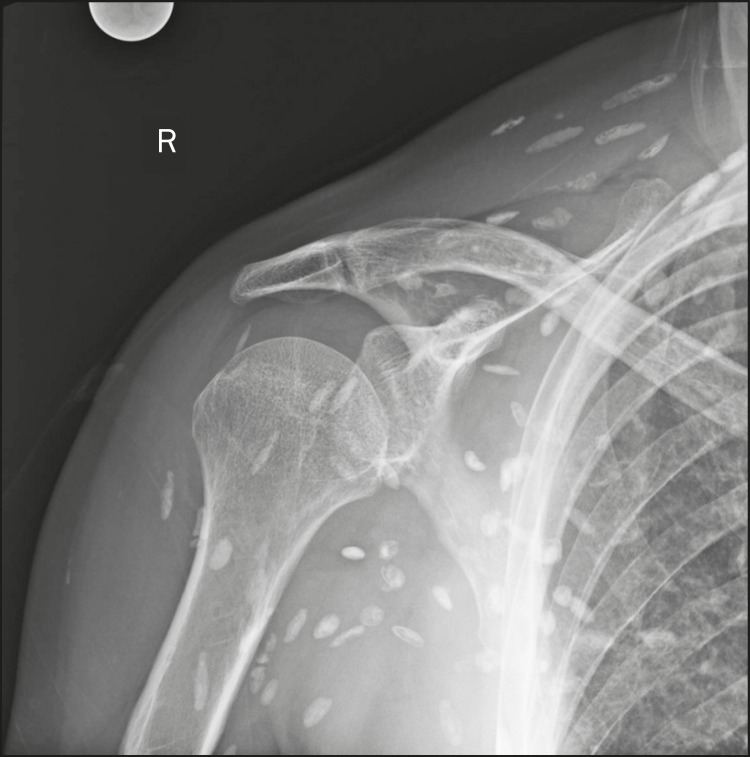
Muscle calcifications in cysticercosis. Frontal X-ray of the shoulder showing multiple dystrophic calcifications diffusely distributed in the muscle bellies, with a typical rice-grain-like morphology.
